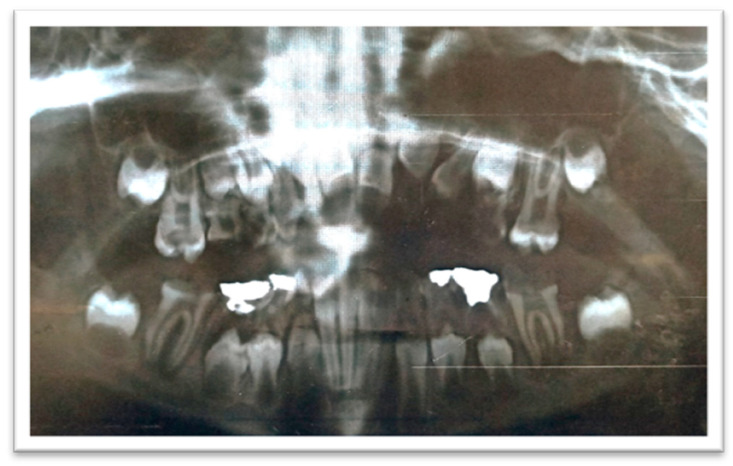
A panoramic radiograph showing teeth #46 and #36 with extremely large carious lesions and periapical radiolucencies, tooth #16 with an extensive carious lesion, and large carious lesions in the upper primary molars.
